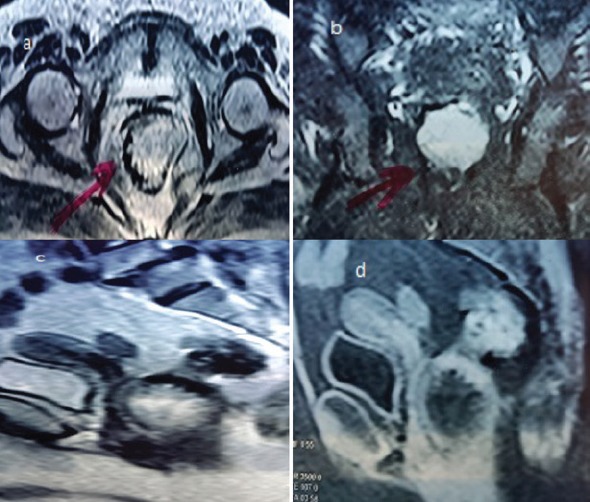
MRI of case two (a) axial section showing large tumour in the left anterolateral wall of the anal canal (b) Coronal section showing no extraluminal extension (c) T1-weighted and (d) T2-weighted sagittal views showing the tumour relation to the posterior vaginal wall.
